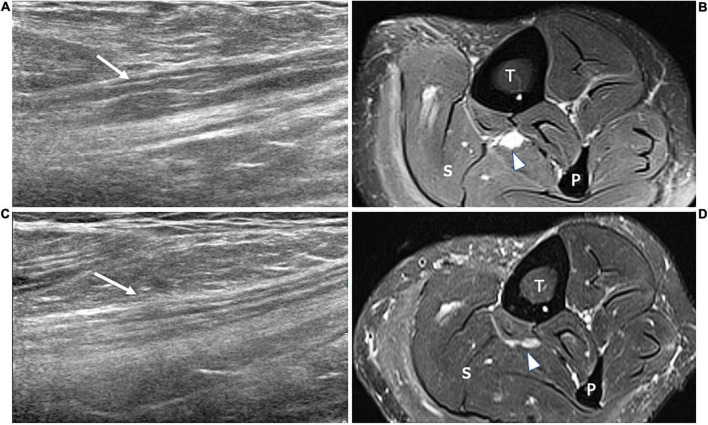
This figure includes two 18 MHz HRUS images (A,C) and two T2-weighted with fat suppression axial MR images (B,D) in a 45 years old female patient affected by rheumatoid arthritis who developed a motor multifocal neuropathy of the tibial nerve after starting a treatment with the anti-TNF-α drug Etanercept. (A) The longitudinal scan of the tibial nerve at the proximal leg shows the focal enlargement of the nerve fascicles (white arrow) to which corresponds the T2-signal hyperintensity (white arrowhead) of the MR image in (B). Both the nerve size and the T2-signal alteration, compatible with intraneural edema ensuing an inflammatory process, result markedly reduced in (B,D). These latter were acquired after 6 months of therapy with immunoglobulin. The findings of this figure highlight the role of HRUS in monitoring the disease activity of dysimmune demyelinating neuropathies. T, tibia; F, fibula; S, soleus muscle.
